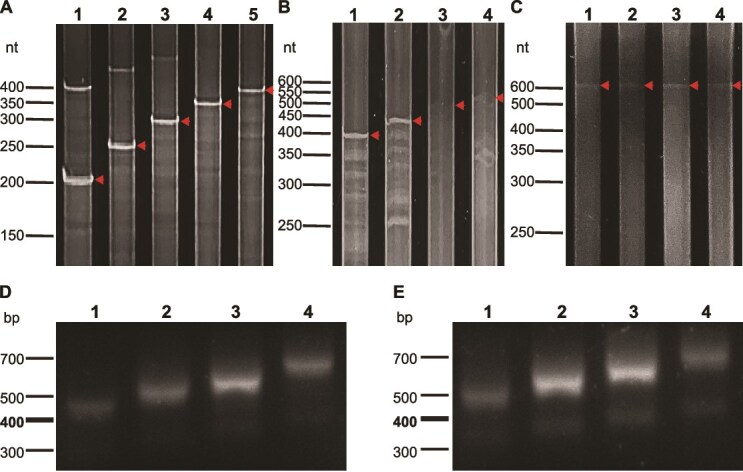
Validation of synthesized ultralong oligonucleotides. (A) PAGE analysis of multiple poly(T) samples, each containing a single fragment of different length (200–400-nt range). Lane 1: 200-nt poly(T) oligo. Lane 2: 250-nt poly(T) oligo. Lane 3: 300-nt poly(T) oligo. Lane 4: 350-nt poly(T) oligo. Lane 5: 400-nt poly(T) oligo. (B) PAGE analysis of synthesized eSTR-containing fragments derived from the *FRA10AC1* gene locus. Each lane represents a distinct fragment with varying lengths ranging from 400 to 550 nt. Lane 1: 400-nt oligo. Lane 2: 451-nt oligo with 17 repeats of eSTR locus. Lane 3: 499-nt oligo with 33 repeats of eSTR locus. Lane 4: 550-nt oligo with 50 repeats of eSTR locus. (C) PAGE analysis of synthesized 600-nt fragments containing 270 CT repeats. Each lane represents the product synthesized by an individual synthesis column (*n* = 4 technical replicates, confirming the stability of the UCOS platform). (D) Agarose gel electrophoresis analysis of biotinylated primer–captured DNA fragments. Lanes 1–4 correspond to the same synthetic oligonucleotide samples as in (B); the captured products were amplified to yield fragments of 400, 451, 499, and 550 nt, respectively. (E) Agarose gel electrophoresis analysis of mismatch repaired DNA fragments. Lanes 1–4 correspond to the same captured products as in (D). Following mismatch repair, amplification was performed using primers harbouring homology arms complementary to the minP-EGFP vector, resulting in extended fragment lengths of 446, 497, 545, and 596 nt, respectively.
